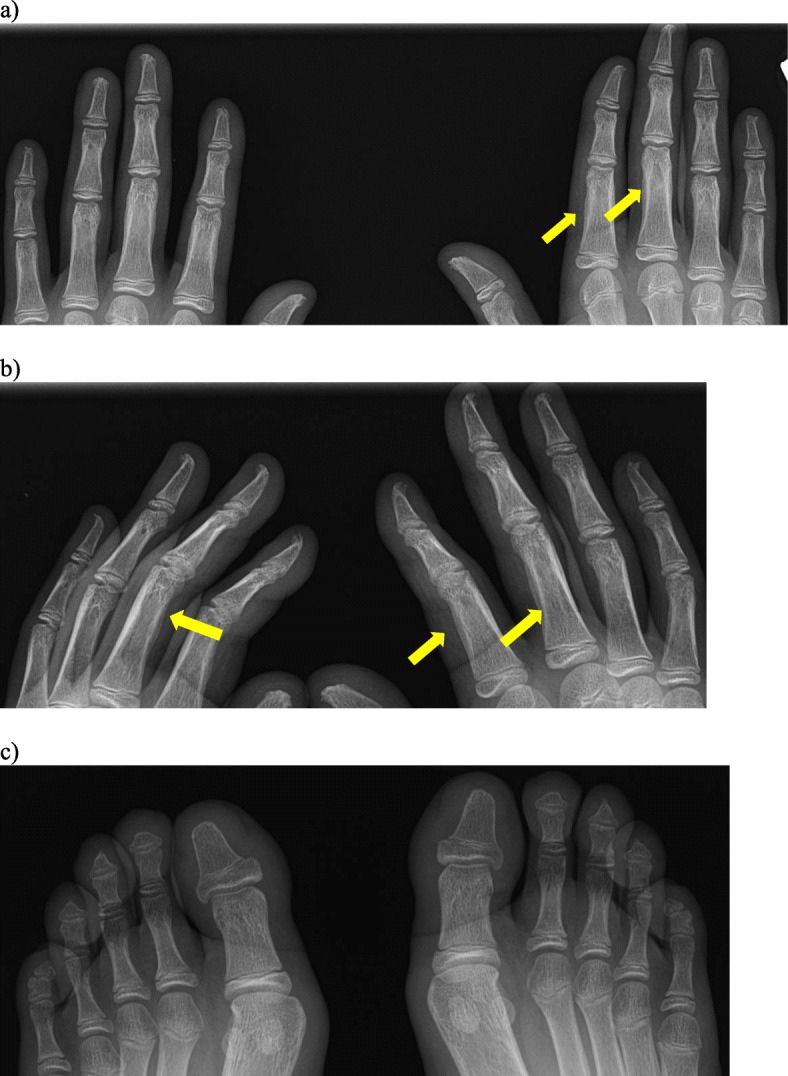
Frontal (a) and oblique (b) radiographs of the hands in a 14 year old male patient with primary hypertrophic osteoarthropathy demonstrate bilateral and symmetric tuft resorption and remodeling. Smooth periostitis involves the proximal phalangeal shafts (arrows). Frontal radiograph of the feet (c) shows smooth tapering of the distal tufts
